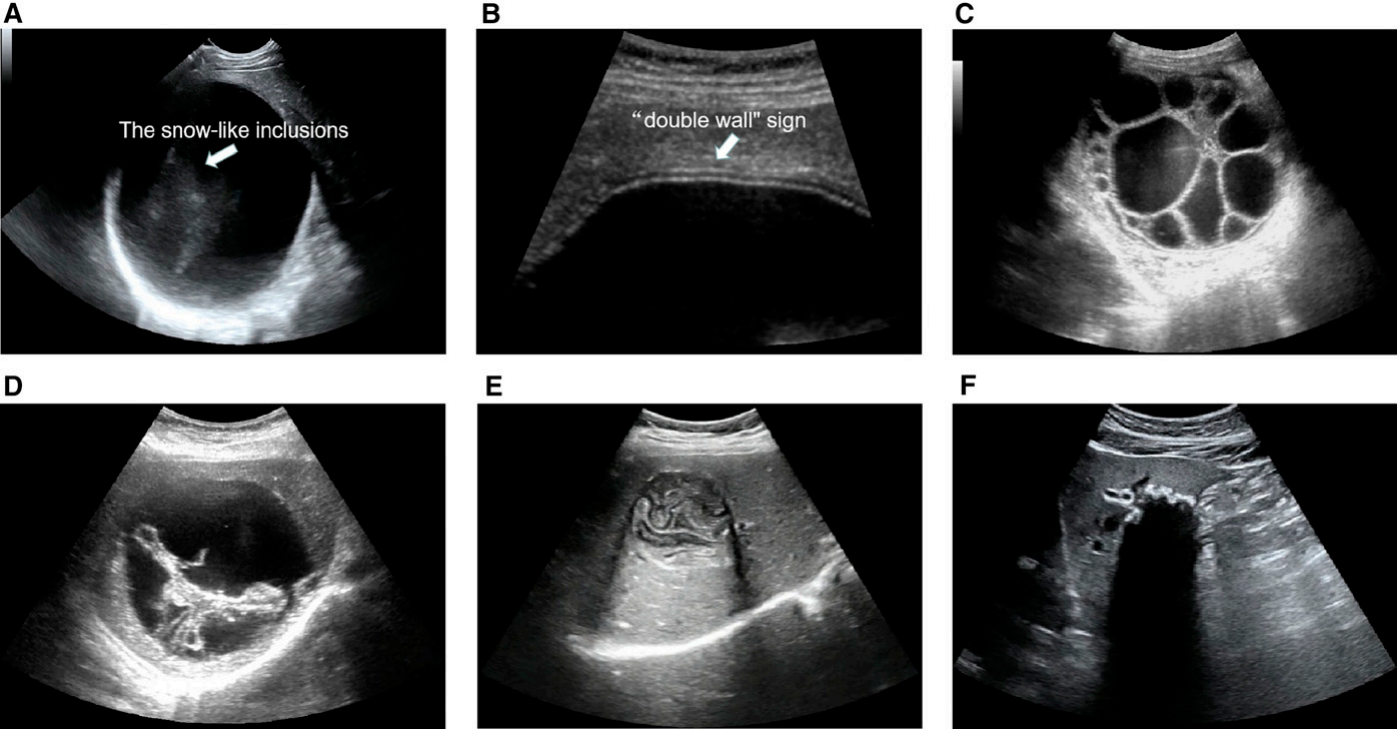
Sonographic images of patients with different hepatic cystic echinococcosis (CE) subtypes. (A) Subtype CE1; the arrow indicates snow-like inclusions, which is also known as “hydatid sand.” (B) The arrow indicates the “double wall” sign. (C) Subtype CE2. (D) Subtype CE3. (E) Subtype CE4. (F) Subtype CE5.
